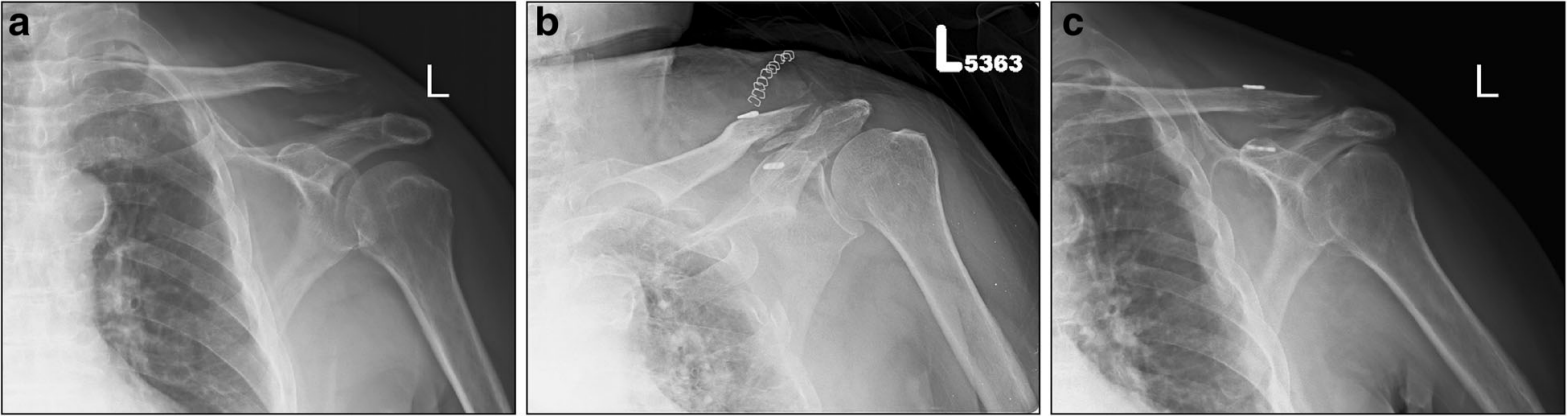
A 32-year-old male with a left distal clavicle fracture (Neer type IIA). During the steps of bone tunnel creation, we changed the trajectory of drilling through the clavicle and coracoid process (a). The endobutton is shown migrating through the inferior surface of the coracoid process due to an occult fracture of the base of the coracoid (b). Posterior-anterior plain radiograph obtained 2 weeks after surgery (c)
